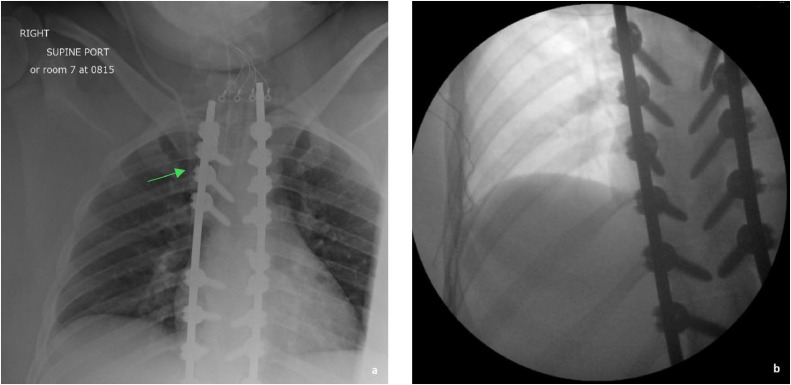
Patient #1. a- a preoperative X-ray showing VP shunt position. b- a postoperative X-ray.
